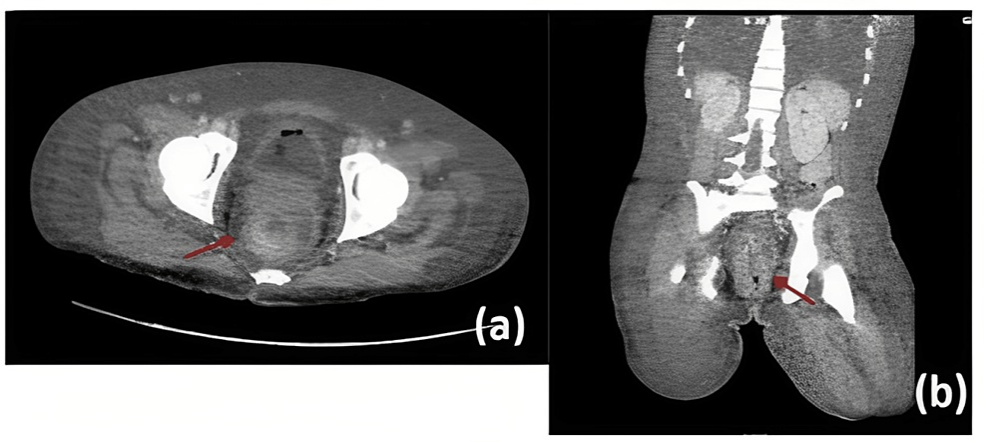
Abdominopelvic CT scan showing inflammatory-looking thickening of the region of the rectum and anal canal (a, b).
